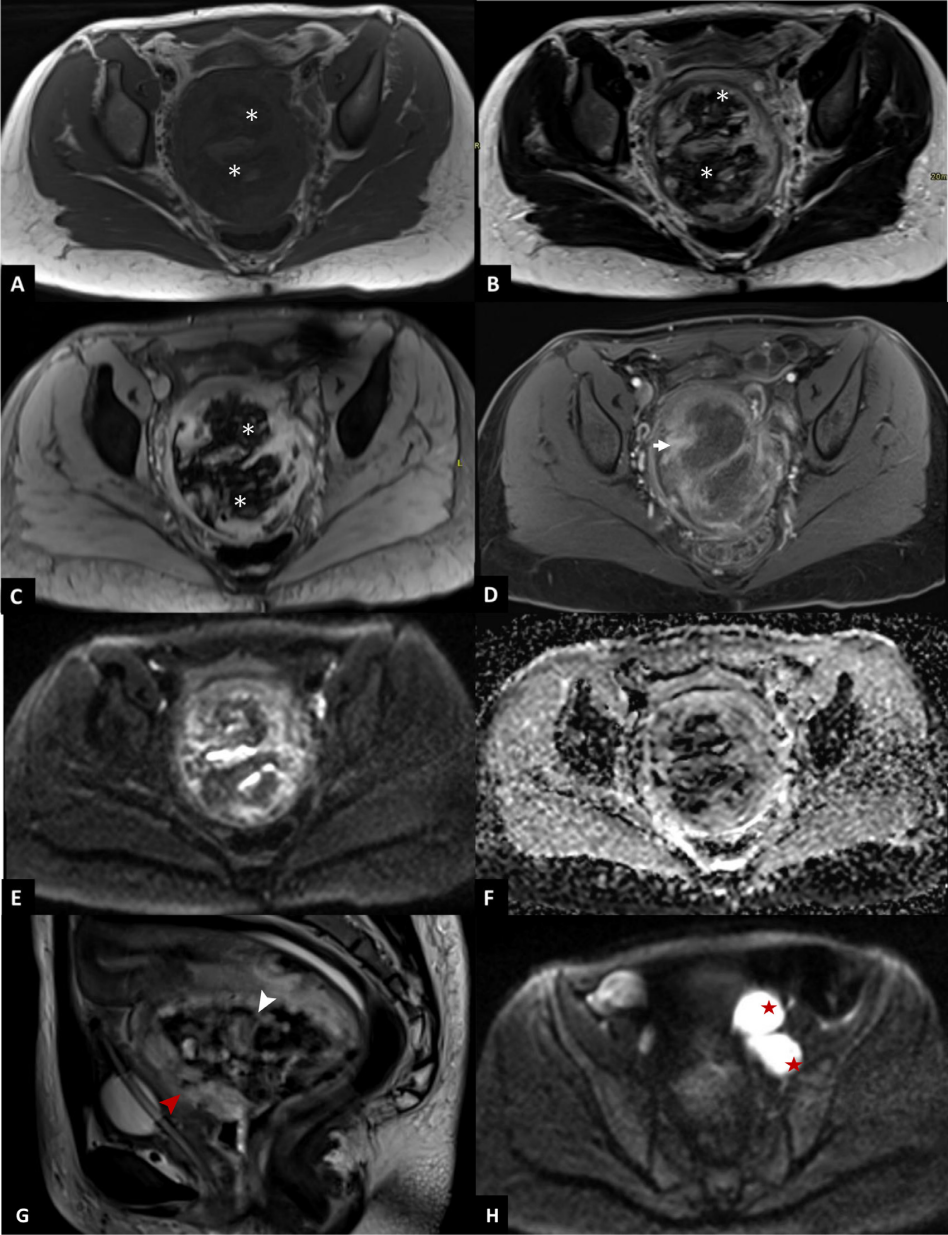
Magnetic resonance imaging (MRI) findings. (A) T1-TSE axial oblique: heterogeneous cervical mass predominantly isointense to muscle with an irregular hypointense necrotic component (asterisks). (B) T2-TSE axial oblique: heterogeneous signal with T2-hyperintense necrotic areas and the same irregular hypointense component (asterisks). (C) GRE T2 axial oblique: blooming of the hypointense component (asterisks), consistent with hemorrhagic products. (D) T1-FS axial oblique post-contrast images show peripheral enhancement (arrows). (E) DWI *b* = 400: minimal diffusion restriction within the solid peripheral component. (F) ADC map: corresponding hypointensity on ADC. (G) T2 sagittal: shows craniocaudal extension with invasion of the uterine isthmus (white arrowhead), uterine body, and posterior bladder wall (red arrowhead), with effacement of the fat plane to the rectum. (H) DWI: enlarged left pelvic lymph nodes (stars).
